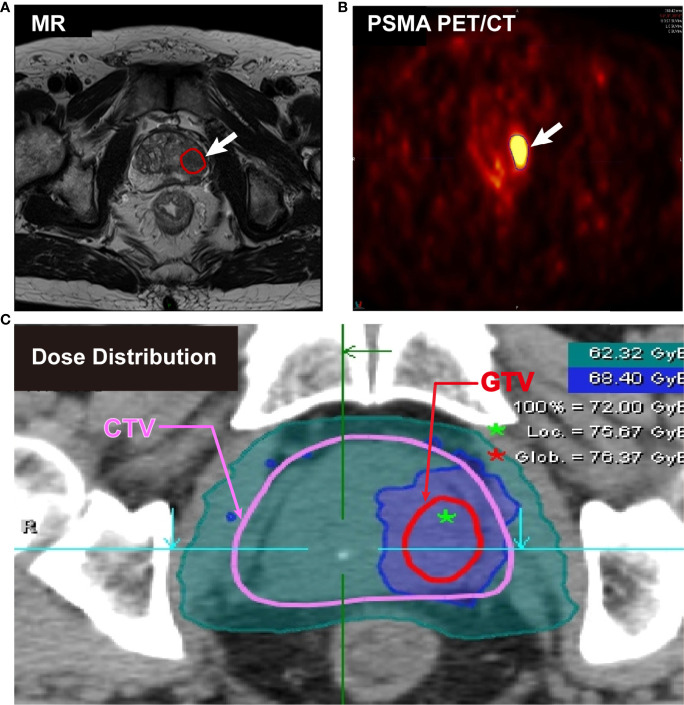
Imaging examination and delineation of tumor volume from a cT3aN0M0 patient. (A) Positive PCa lesions on MR image. (B) High uptake positive lesions on ^68^Ga-PSMA PET/CT image. (C) Clinical target volume (CTV) (red) and gross target volume (GTV) (pink) for simultaneous integrated boost (SIB) on planning CT.
